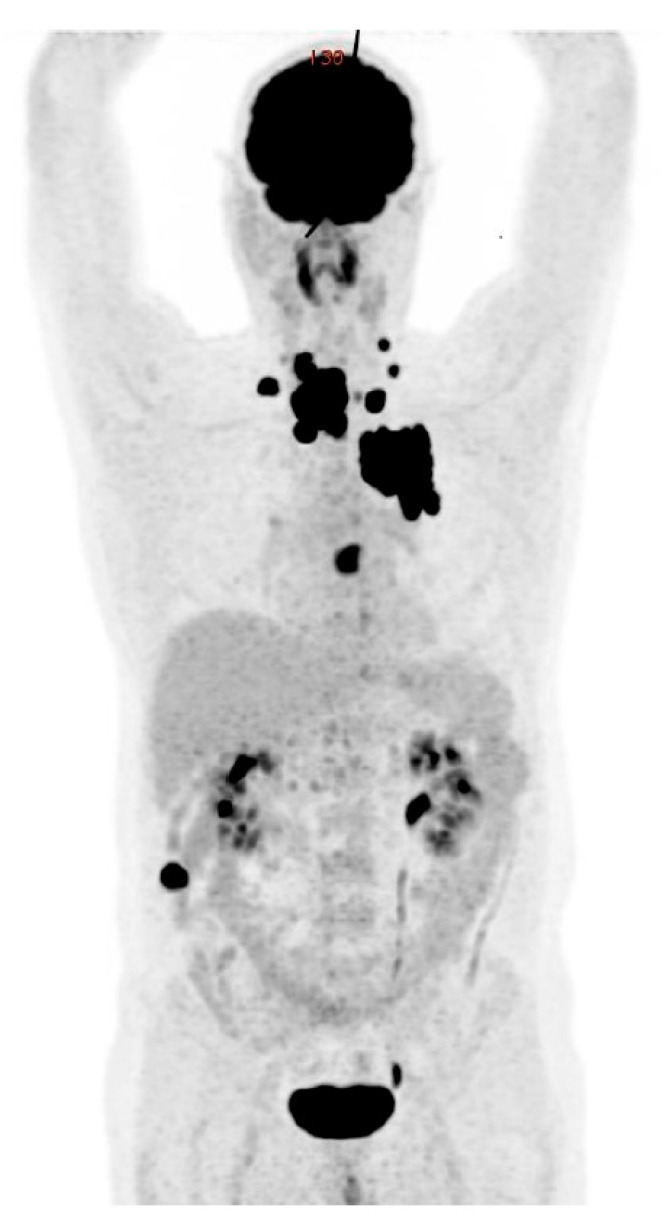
[^18^F]FDG-PET/CT: anaplastic thyroid cancer with diffuse loco-regional invasion, lymph node, and distant metastases (lung, heart, and bone).
